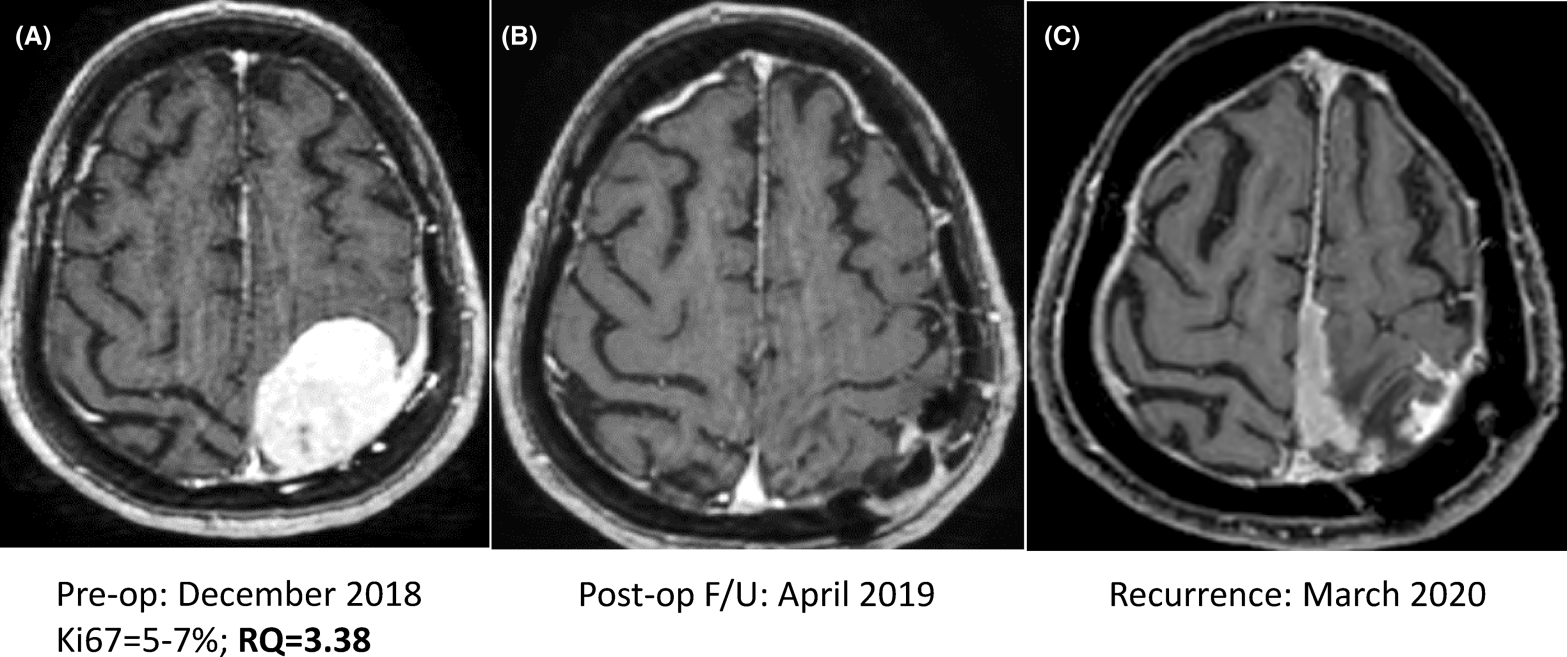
Gadolinium enhanced MRI studies of a 58‐year‐old female with WHO 1 meningioma with a preoperative elevated level of circulating exosomal hTERT transcripts. (A) Preoperative imaging. The serum relative quantification (RQ) value for telomerase expression prior to surgery was above normal level of healthy controls. (B) Postoperative follow‐up (F/U) imaging confirms gross total resection of the Grade 1 meningioma. (C) Routine surveillance imaging 15 months after surgery reveals tumor recurrence.
